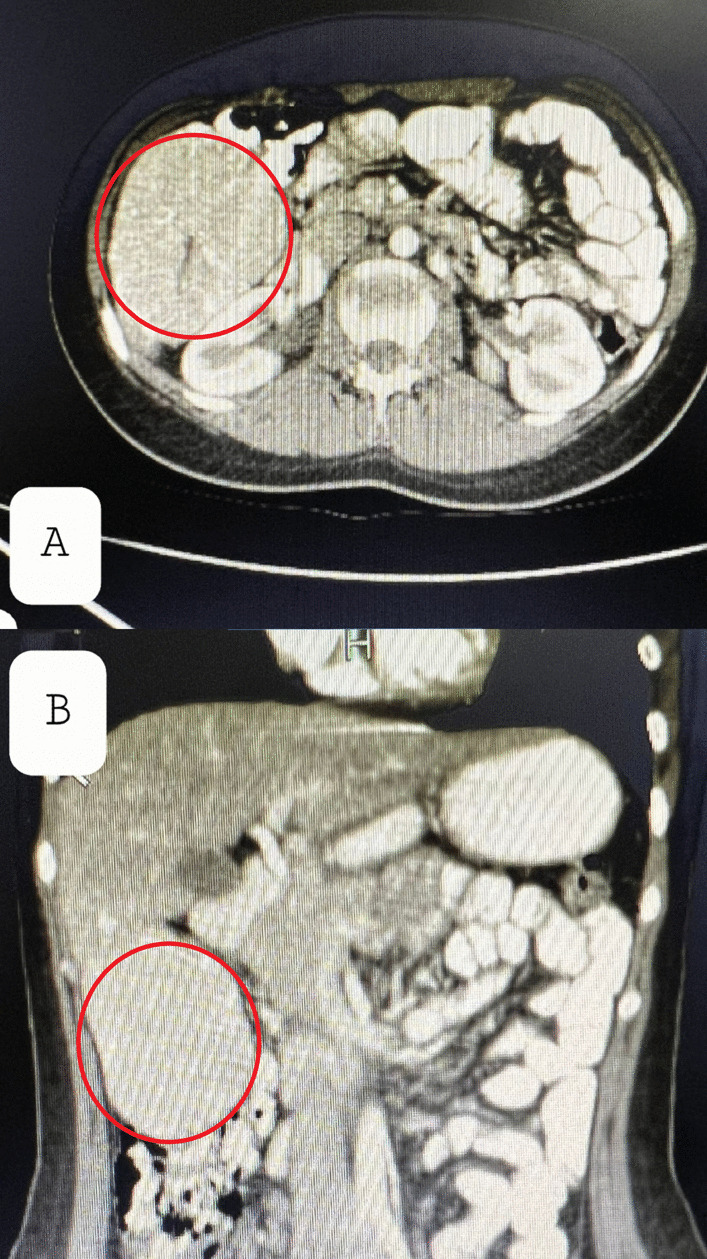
Computed tomography images. The red circles represent the FNH, which is demonstrated as a well-circumscribed mass emanating from the right lobe of the liver on axial (A) and coronal (B) planes
